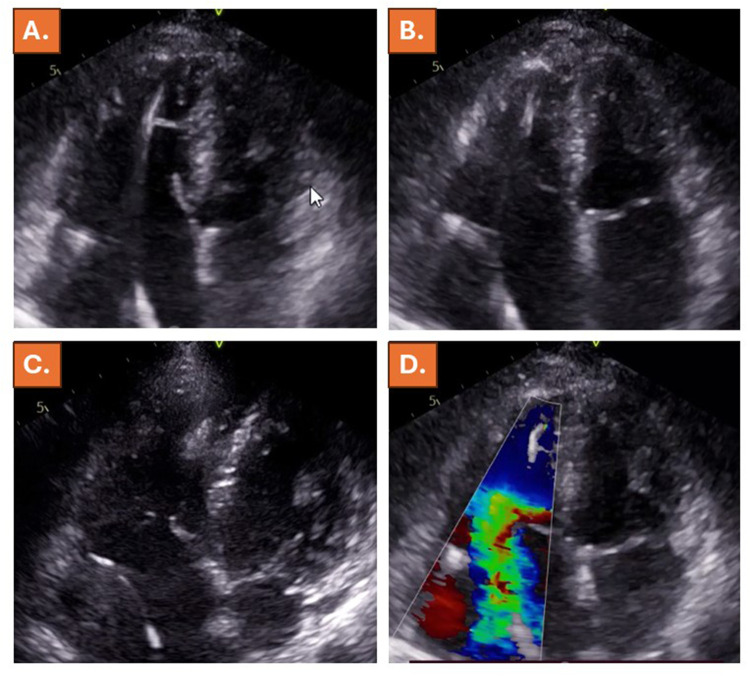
Normal diameters and function of the LV and LA but enlargement of the RV, reduced RV-function and severe RA enlargement by echocardiography. Systolic image in (A), diastolic image in (B), coaptation gap of 6 mm in (C) and torrential TR by Doppler ultrasound shown in (D).
